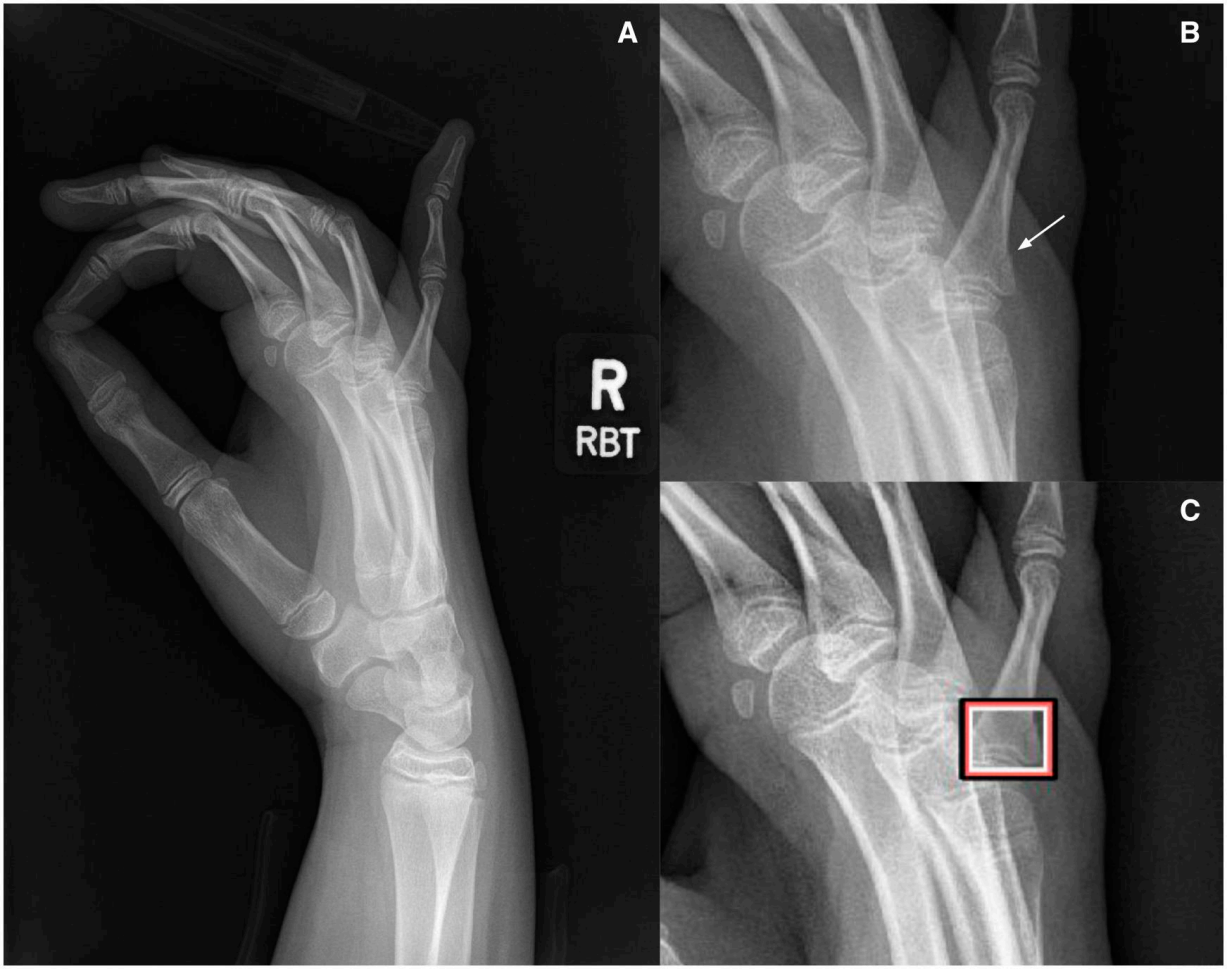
A lateral paediatric hand radiograph (A) with a Salter Harris type II fracture of the base of fifth proximal phalanx (B, white arrow). The fracture was detected by the AI tool (C). The fracture was missed by 11 readers in the unaided exam, but detected by 14 readers in the aided exam with support from the AI tool.
